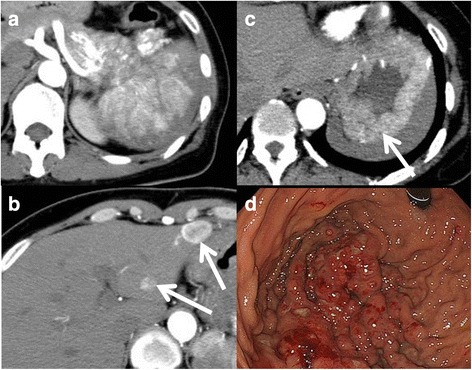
Abdominal contrast-enhanced CT findings and upper endoscopy findings. CT showing a hypervascular tumour in the tail of the pancreas, infiltrating the spleen and the splenic vein (a). Multiple hypervascular tumours in the liver (b). Hypervascular tumour in the upper part of the stomach (c). Upper endoscopy showing a big tumefactive lesion in the fornix and greater curvature (d)
